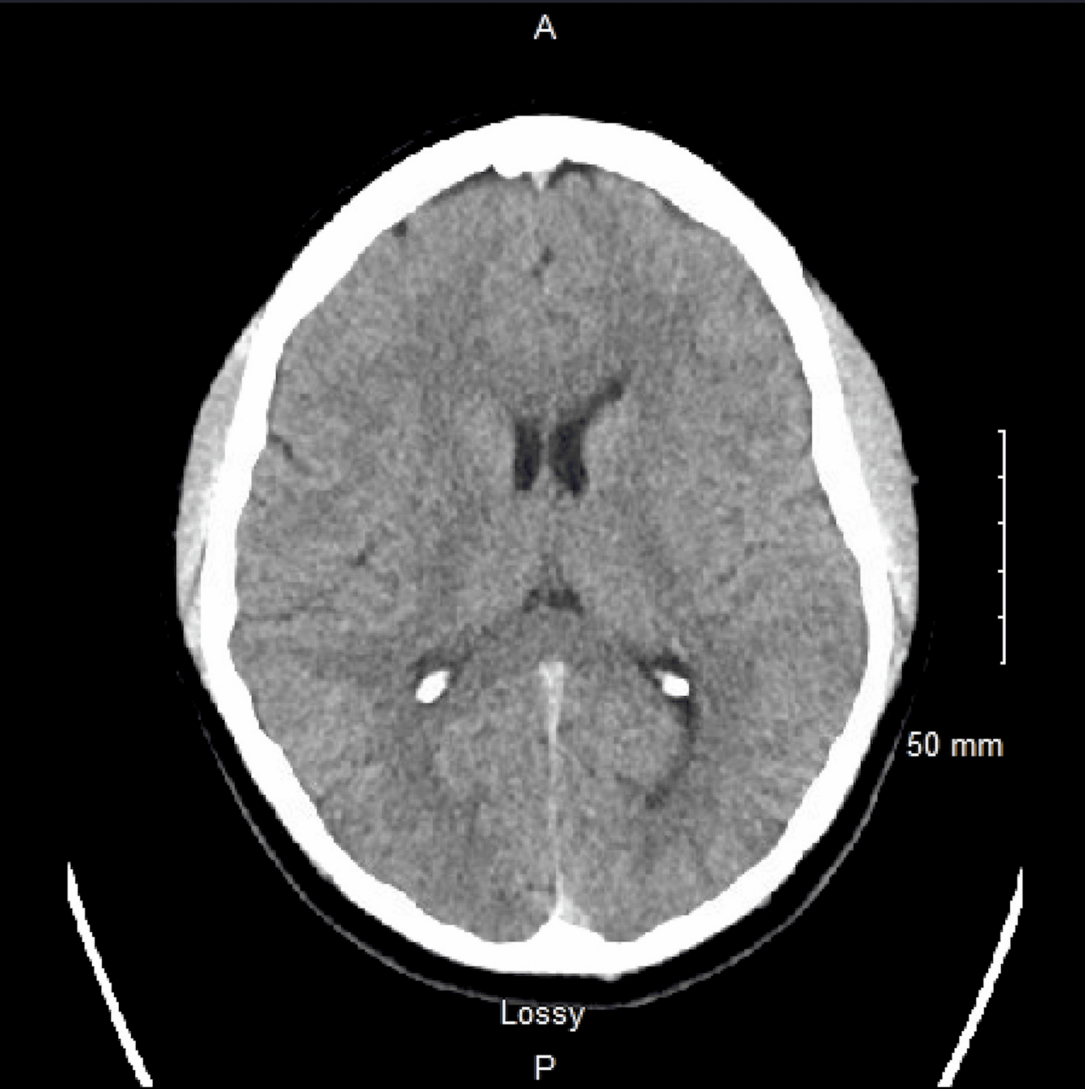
Head CT showing no acute abnormalities
